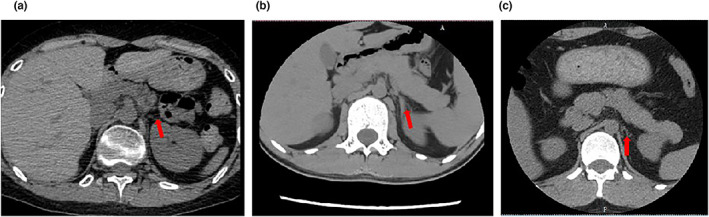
CT images the X‐linked adrenal hypoplasia congenita patients. Patient 1: adrenal atrophy (a). CT images Patient 2: adrenal with increased thickness (At the age of 17) (b) Patient 2: adrenal atrophy (At the age of 25) (c). The position of adrenal was labeled by the red arrow.
